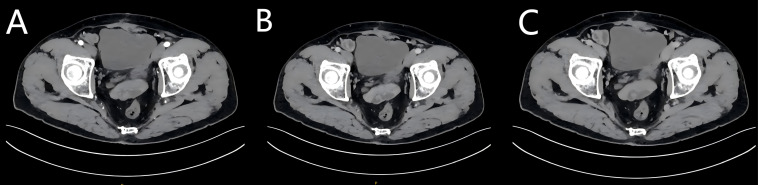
Enhanced CT examination at the 6-month postoperative follow-up. The images show normal postoperative manifestations with a uniform enhancement. (A) Arterial phase; (B) Venous phase. (C) Delayed phase.
